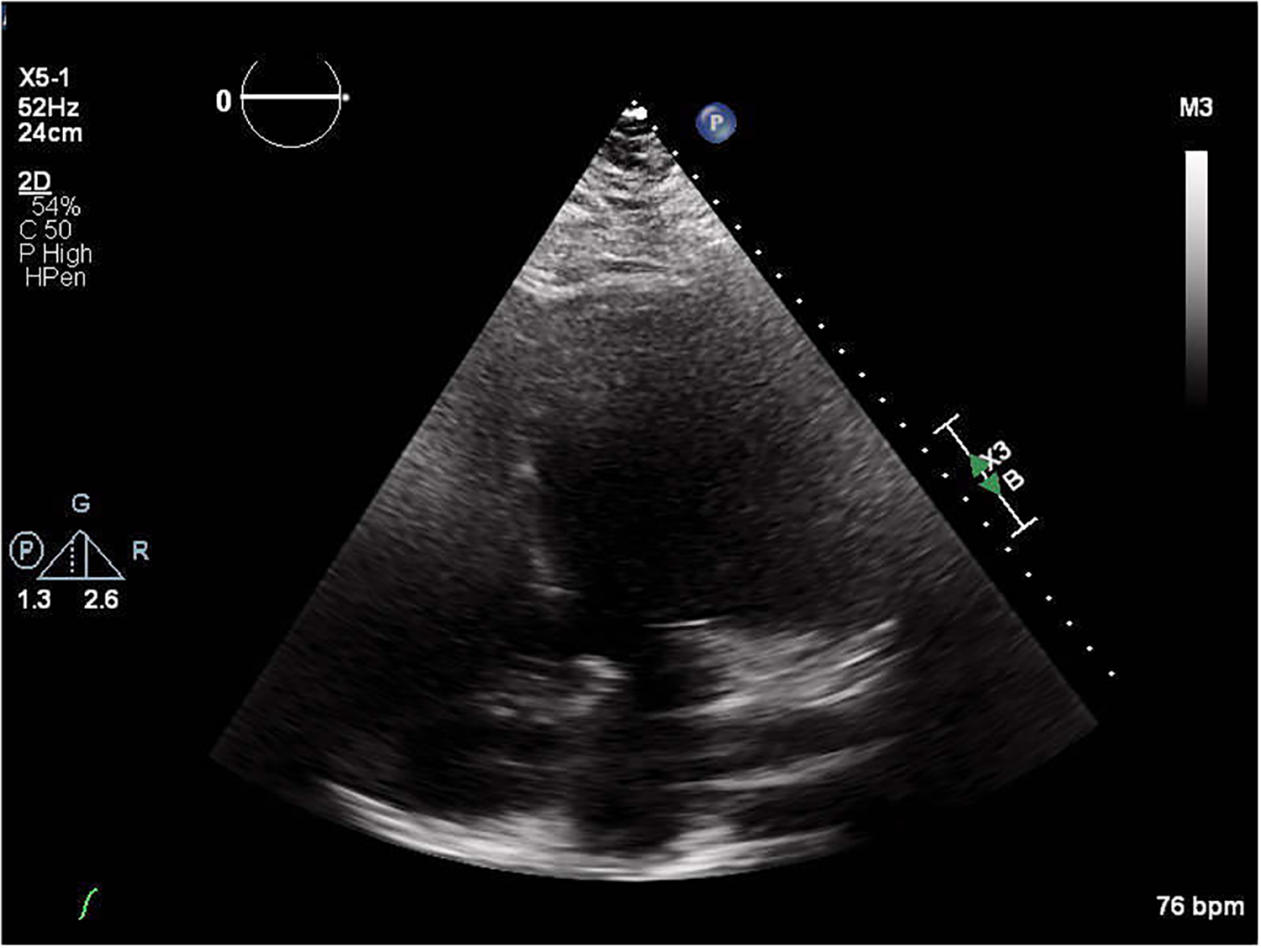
Echocardiograph of an LVAD patient depicting the result of poor acoustic windows.
